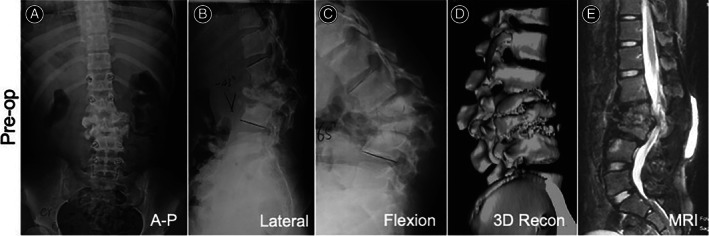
Initial radiological assessment showed lumbar kyphosis. (A) Anterior–posterior (A–P) view of the thoracic‐lumbar spine. (B) Kyphotic angle of 43° at the L2, L3 segment. (C) Kyphotic angle of 65° at flexion state; destructive appearance in centrums from L1 to L4. (D) Three‐dimensional reconstruction shows destruction of centrums. (E) MRI shows compression of the dual sac and stenosis of central canal. Figure 1A, B, E are cited from our previously published report on this case.^5^
